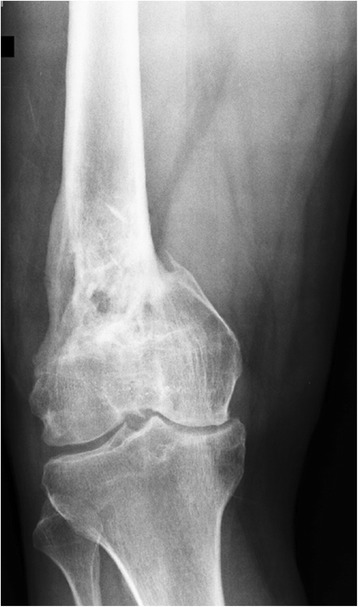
Anteroposterior radiograph of the right femur 2 year after the subcutaneous emphysema of the leg
